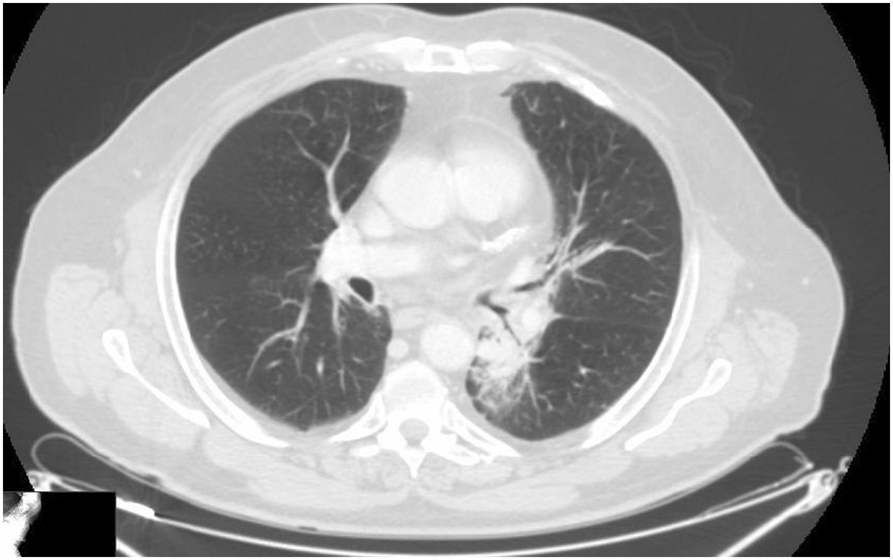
Computed tomography scan performed before starting erlotinib treatment.
